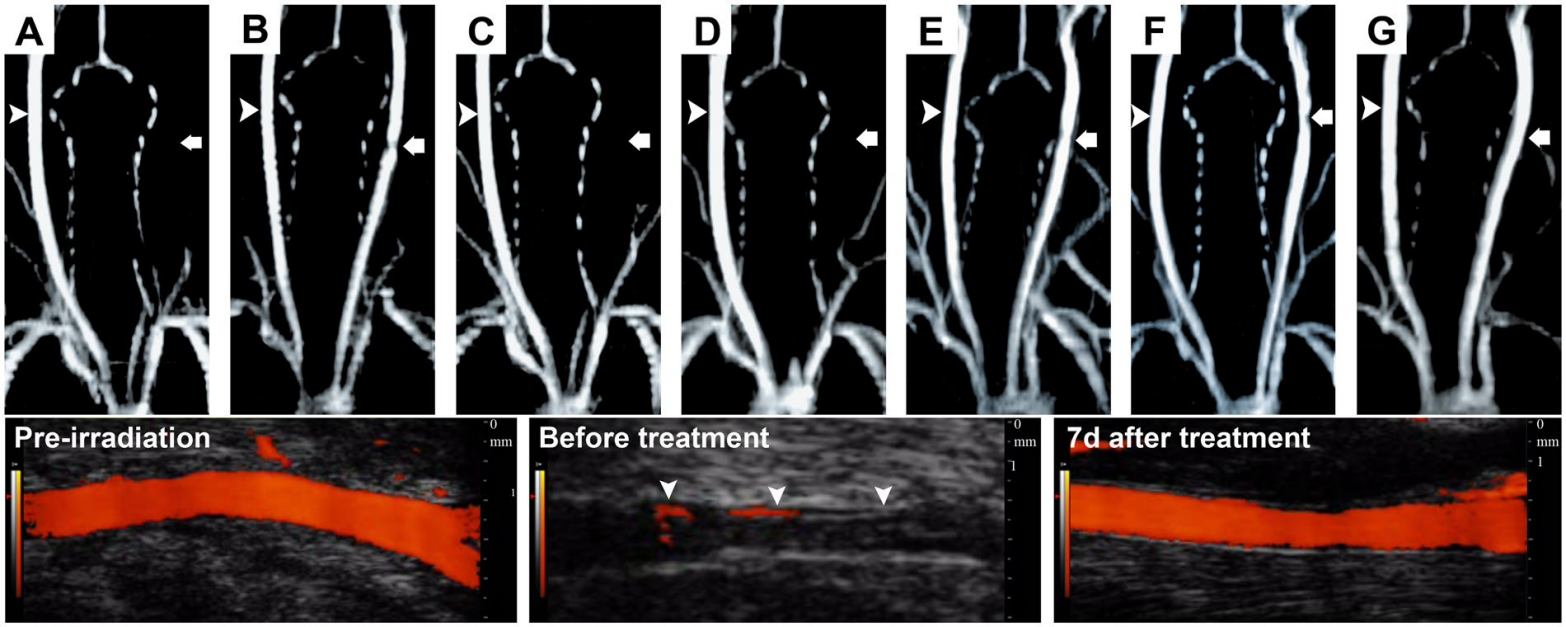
MR angiographic findings at 7d in each treatment group. The left common carotid artery (CCA) is occluded in groups A, C and D. However, left CCA shows some degree of stenosis in groups B, E, and F. In group G, there is complete recanalization with minimal residual stenosis. The carotid ultrasound examination demonstrates the change in group G which shows the normal CCA before LED irradiation (pre-irradiation), after irradiation, complete occlusion of CCA before rt-PA treatment (before treatment, arrowheads indicate vessel wall), and recanalization of occluded CCA at 7d after rt-PA treatment (7d after treatment). In A-G, the arrow indicates the injured left CCA, and arrowhead indicates the normal right CCA.
